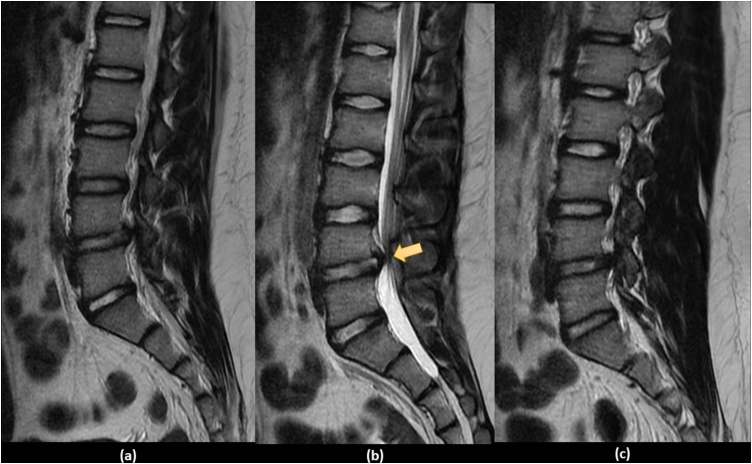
Sequential sagittal T2 weighted MRI images showing large L4 L5 disc herniation: (a) left paramedian sagittal view, (b) midsagittal view and (c) right paramedian sagittal view.
